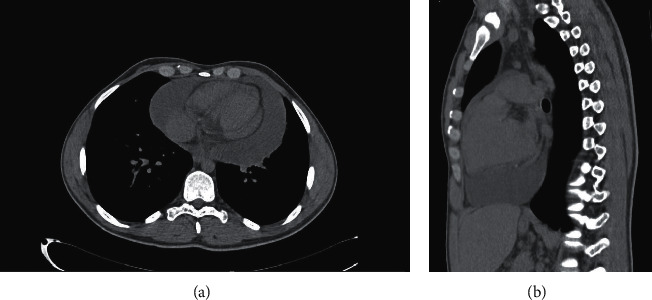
Axial (a) and coronal (b) sections of a nonenhanced CT chest showing pericardial effusion.
